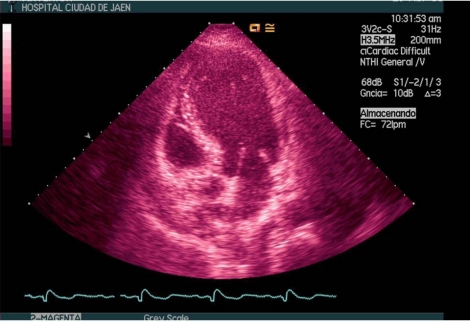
Echocardiographic image of a patient in septic shock secondary to fecaloid peritonitis. The left ventricle shows a pathological remodeling similar to that generated in an anterior wall acute myocardial infarction.
